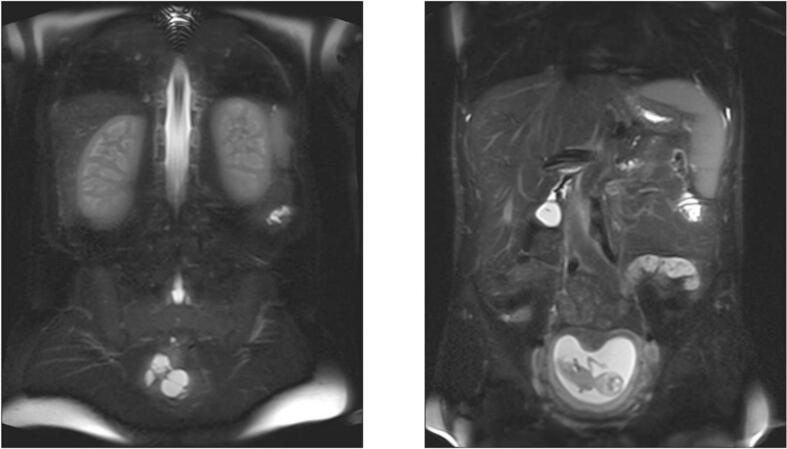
II) July 2018, Abdomen and pelvis, Coronary slices (frontal plane), Sequence: T2-weighted MRI sequence, no contrast-enhancement.
